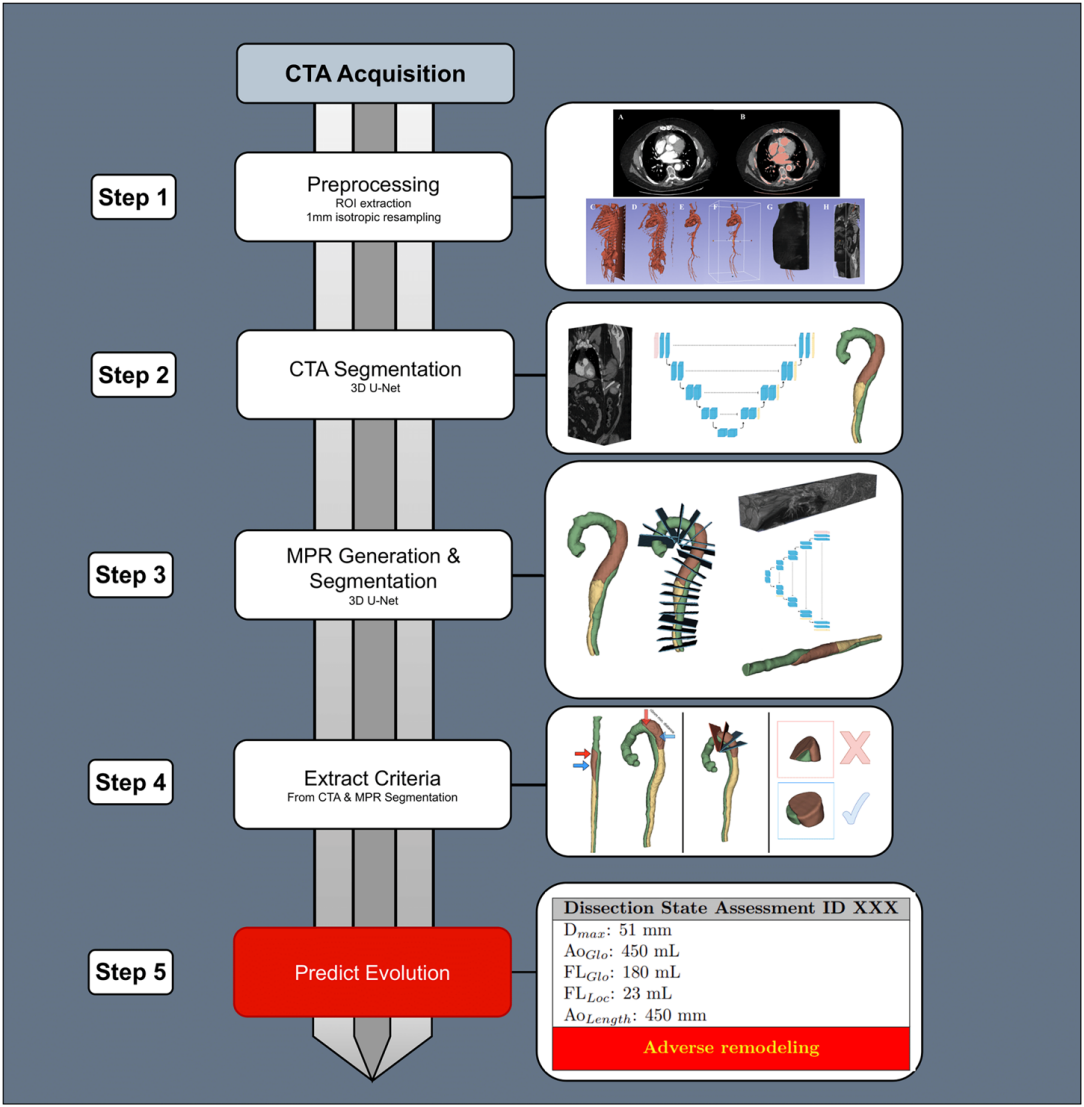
Model description in five steps. CTA, computed tomography angiography; ROI, region of interest; MPR multiplanar reconstruction; *D*_max,_ maximal aortic diameter; Ao_Glo_, global aortic volume_;_ Ao_Length_, total aortic length; FL_Glo_, global false-lumen volume; FL_Loc_, local false-lumen volume
